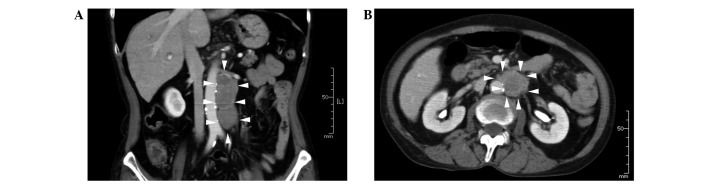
Contrast computed tomography (CT) images using lopamiron multiplanar reconstruction. (A) Colonal plane at the level of the aorta. (B) Horizontal plane at the level of the kidney veins. CT examination revealing an abnormal bulky tumor pressing on the left kidney artery along the left side of the aorta between the left kidney artery and the bifurcation of iliac arteries.
